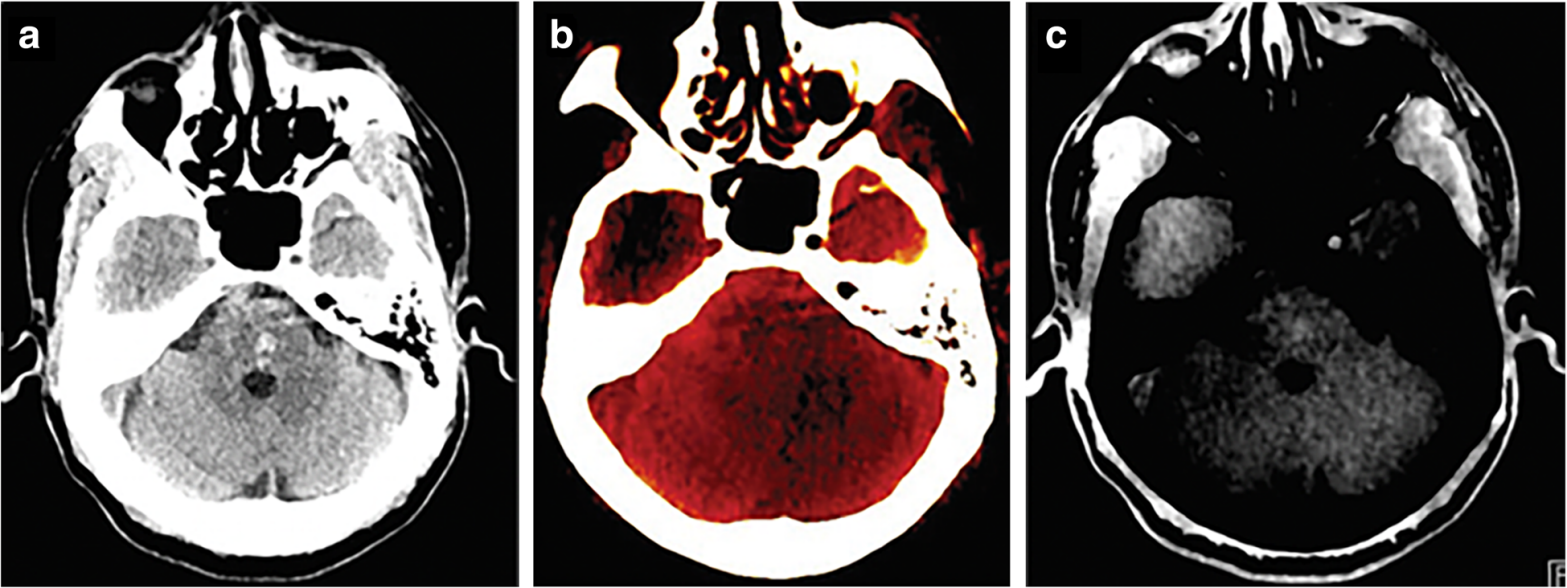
Head dual-energy computed tomography scan shows focal hyperattenuation in the pons, classified as indeterminate at standard grayscale series (a). This 58-year-old patient had a history of hypertension and diabetes and presented to the emergency department with right arm tremor, blurry vision, and increased systolic blood pressure. On the calcium-overlay image, the focal hyperattenuation in the pons was not apparent (b). On the virtual non-calcium image (c), the focal hyperattenuation in the pons manifests as a focal area of high attenuation, compatible with hemorrhage. From Hu et al. [17]
